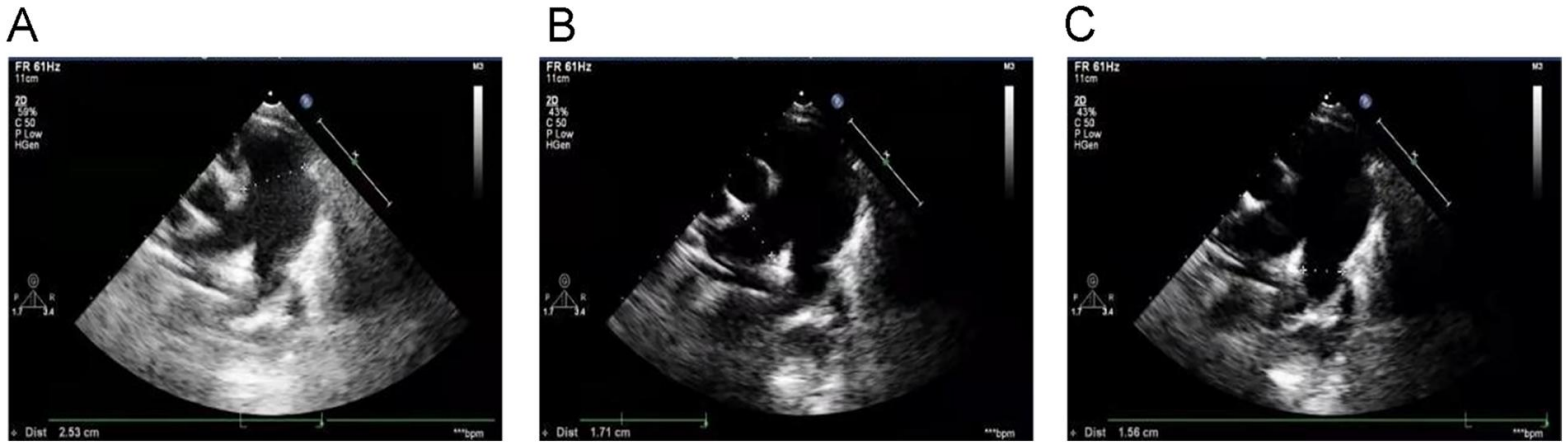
Echocardiography with TDI and EF measurement: (A) main pulmonary artery diameter (25 mm), (B) right pulmonary artery diameter (17 mm), (C) left pulmonary artery diameter (16 mm).
